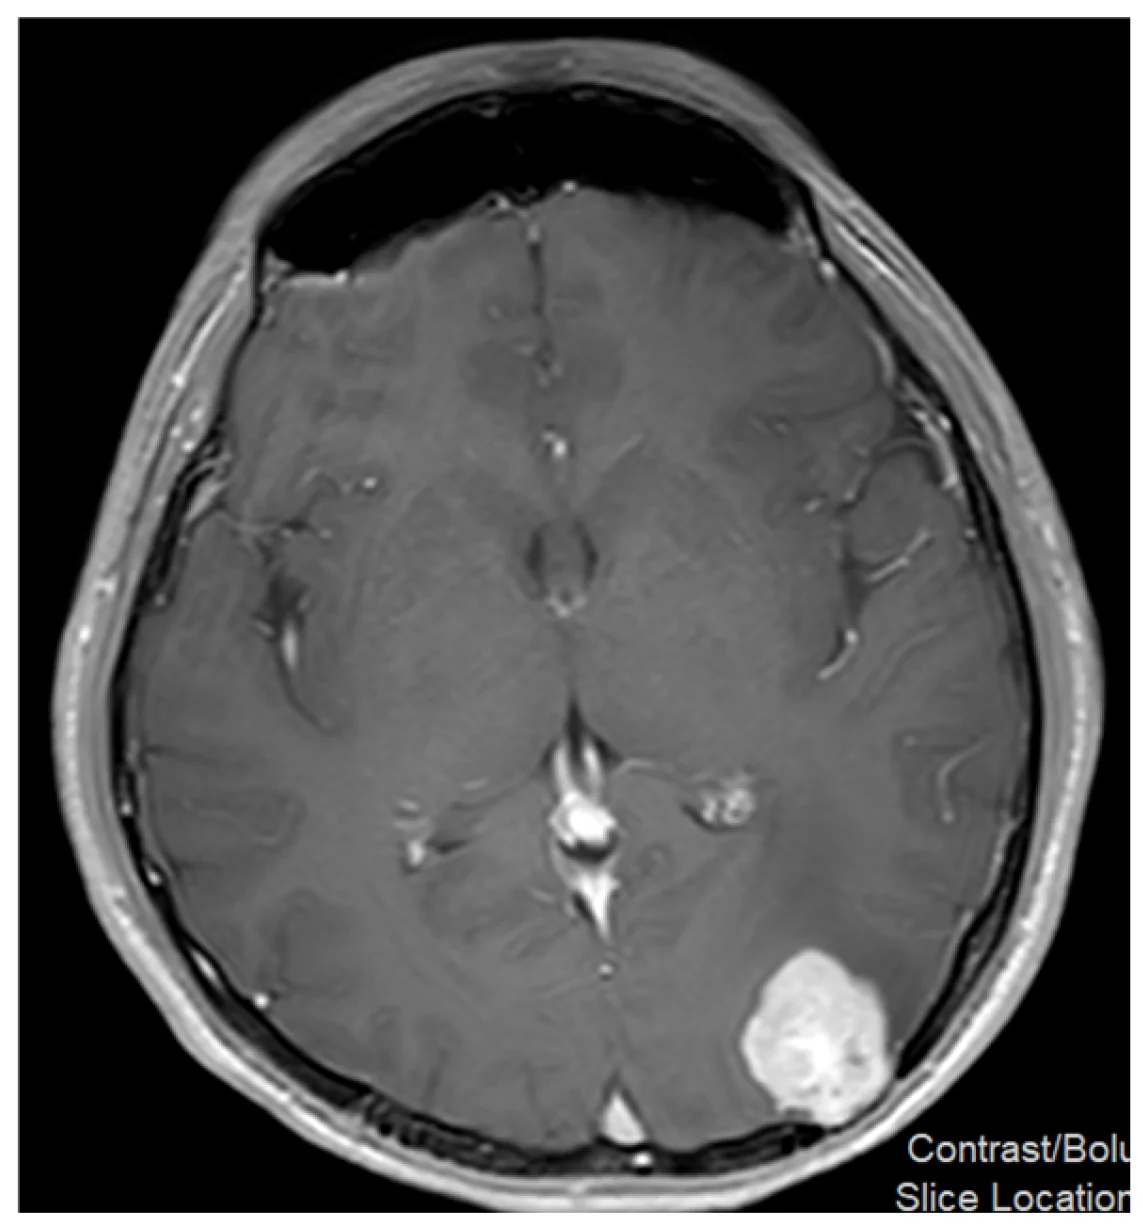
Preoperative brain MRI showing a lobulated, peripherally located, contrast-enhancing intra-axial mass in the left occipitoparietal region.
